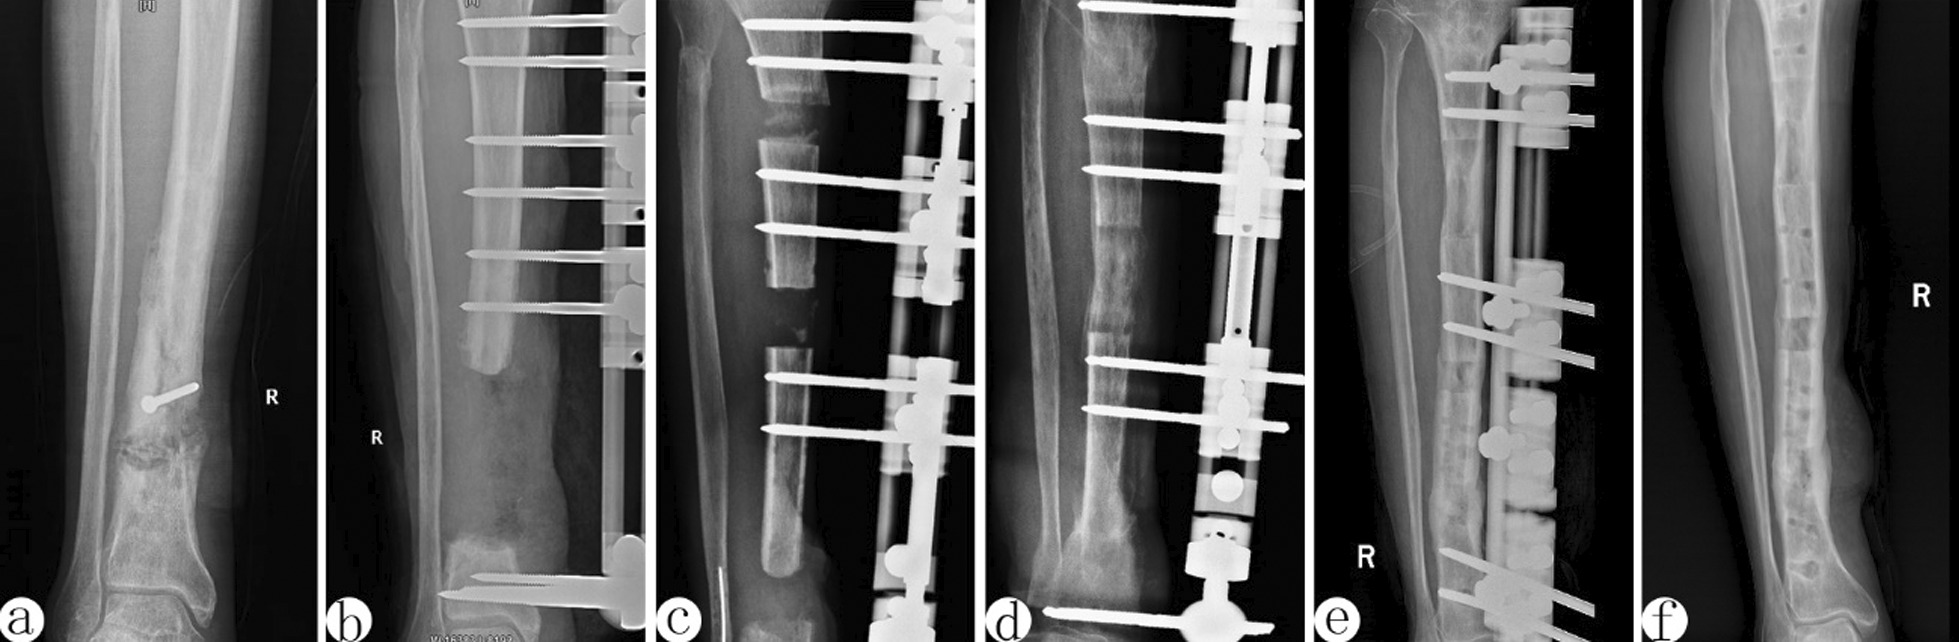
A 47-year-old female patient with posttraumatic osteomyelitis the right tibia and treated using Orthofix external fixator trifocal bone transport from proximal to distal. a X-ray graph before treatment. Lesions and internal fixator were on the sides of the middle and lower part of the tibia. b Segmental defect of the left tibia after debridement on X-ray graph. Excision of infection bone with 12 cm defect and application of Orthofix external fixation with trifocal bone transport. c The X-ray graphs at 40 days after surgery. The force line was available. d Bone healing of the osteotomy line was evident at 4 months. e Bone transport was completed with good regenerate consolidation and docking union was achieved and evaluated on the view of X-ray at 6 months. f Orthofix external fixator was removed with excellent bone union shown on the view of X-ray at 8 months after operation
